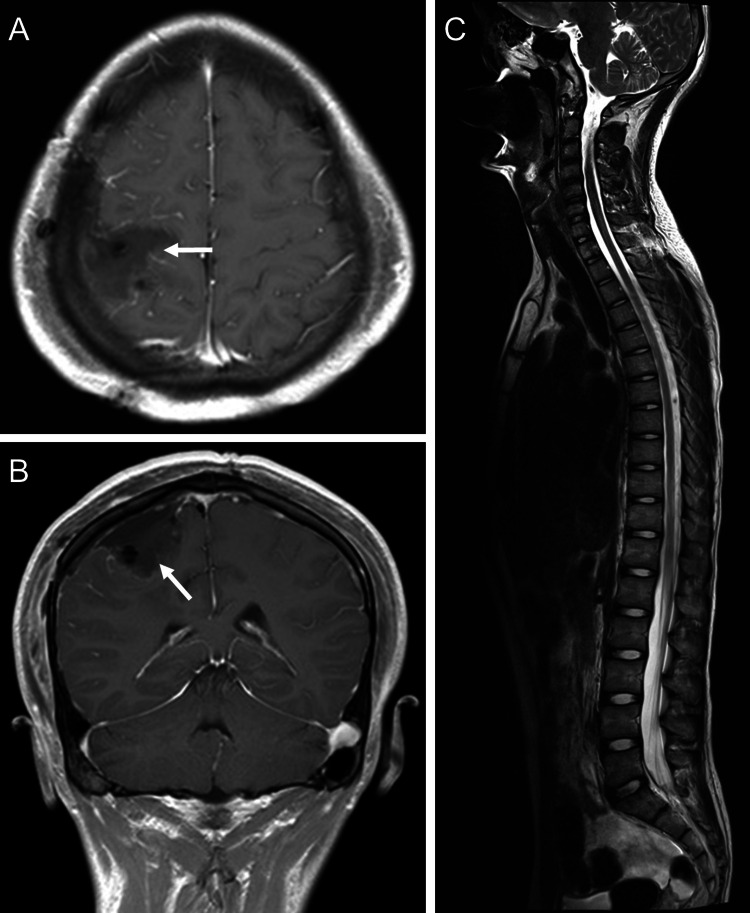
Postoperative MRI images (A, B) Post-surgical MRI in an axial T1-weighted section with contrast shows total tumor resection (white arrows). (C) Craniospinal MRI in a sagittal T2-weighted section without evidence of metastatic lesions.
